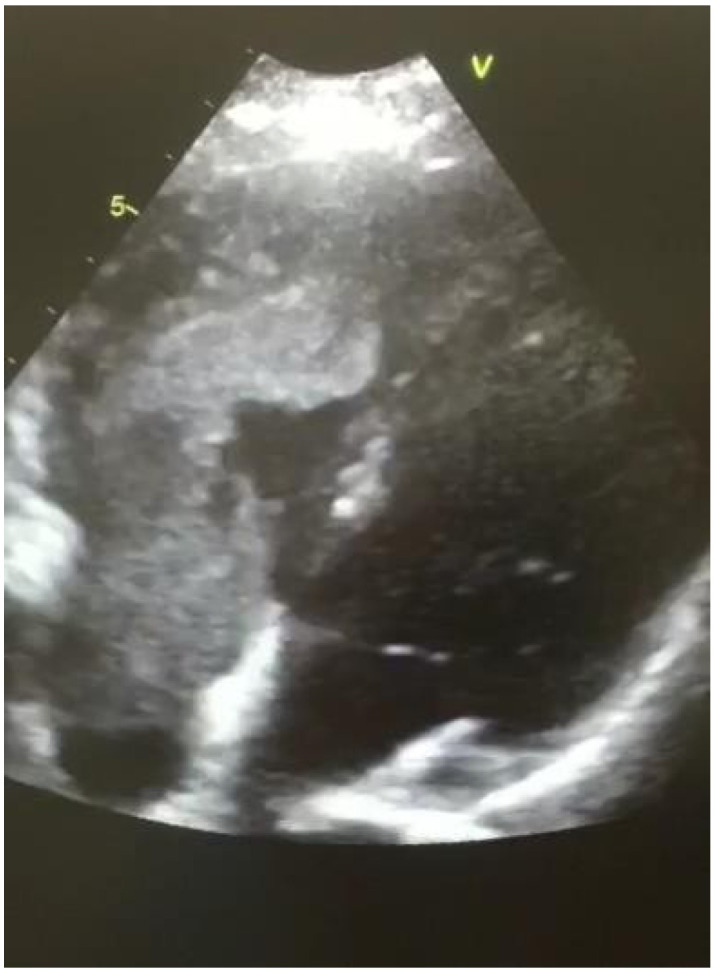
Image of transthoracic echocardiography showing the presence of a massive embolus in the right atrium and through the tricuspid valve.
